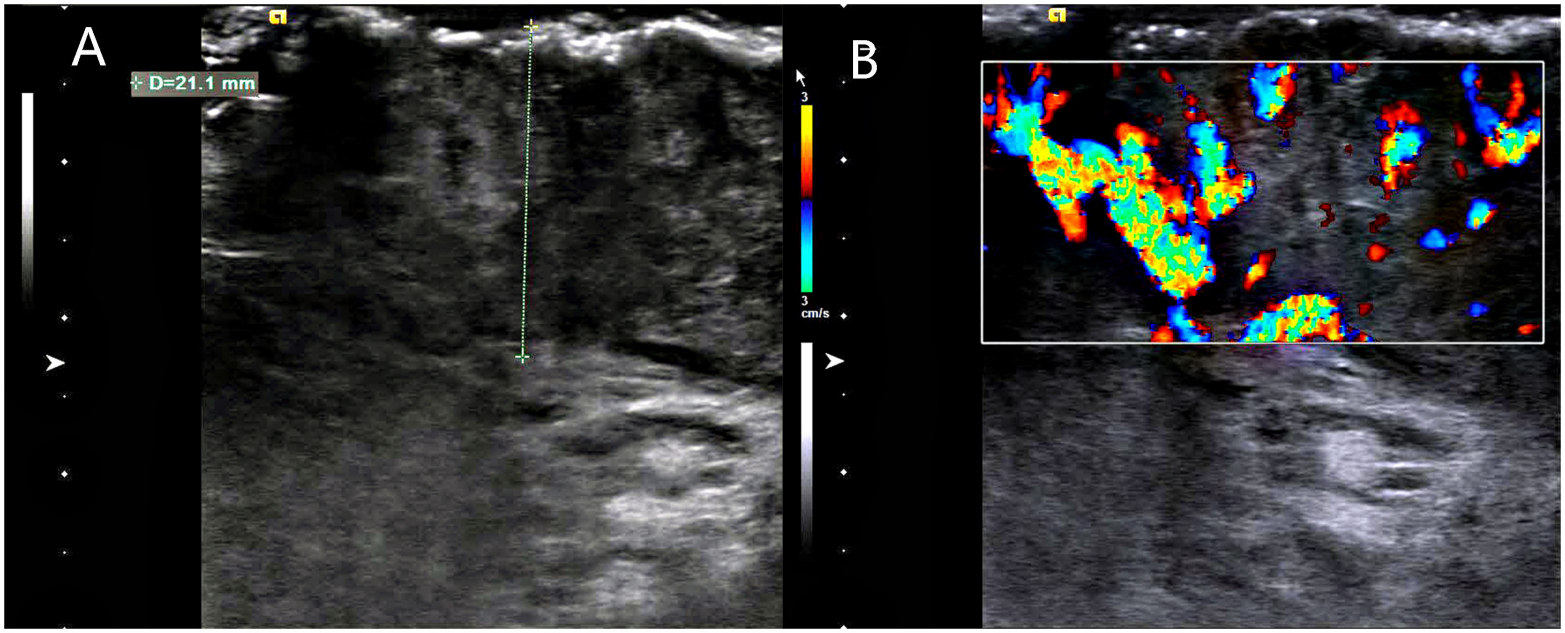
Breast ultrasonography. (A) A large mass was seen on the surface of the right chest wall, the thickest part of which was about 21.1mm, and the inner part of the mass was uneven and hypoechoic. (B) color Doppler flow imaging(CDFI): abundant blood flow signal is seen inside the mass.
